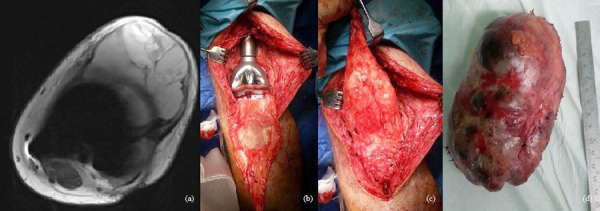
a) Chondrosarcoma G3, local reoccurrence in patella after 1year (MRI); b-c) 2017, we performed resection and transplantation of patella from a donor (operating room view); d) resected tumor – patella. Chondrosarcoma G3.
